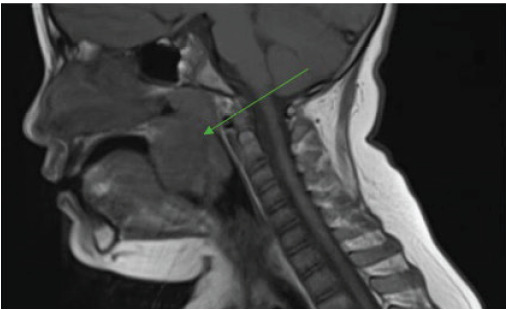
Magnetic resonance imaging sagittal view depicting oropharyngeal mass (arrow).
